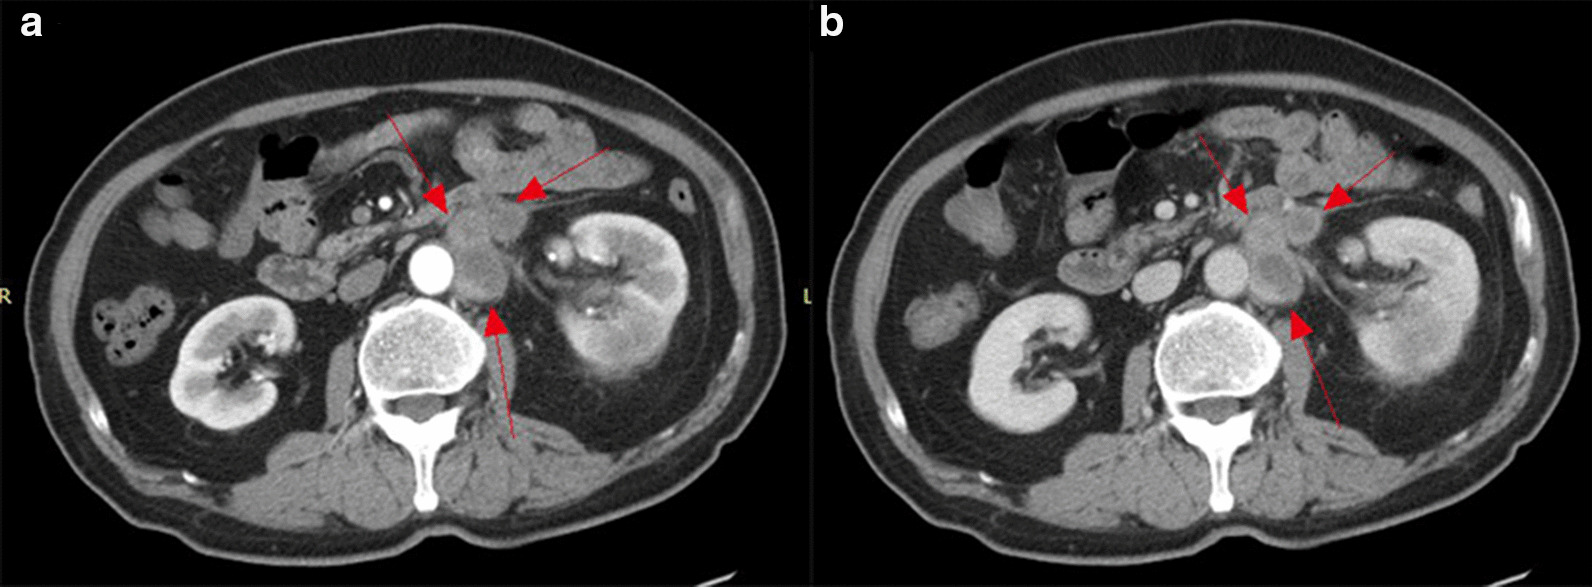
Case 6: a axial enhanced CT image shows left renal CDC. Axial arterial phase image showing multiple retroperitoneal enlarged lymph nodes with marginal mild enhancement (arrows). b Axial delayed phase image shows further strengthening, which is similar to renal CDC (arrows)
